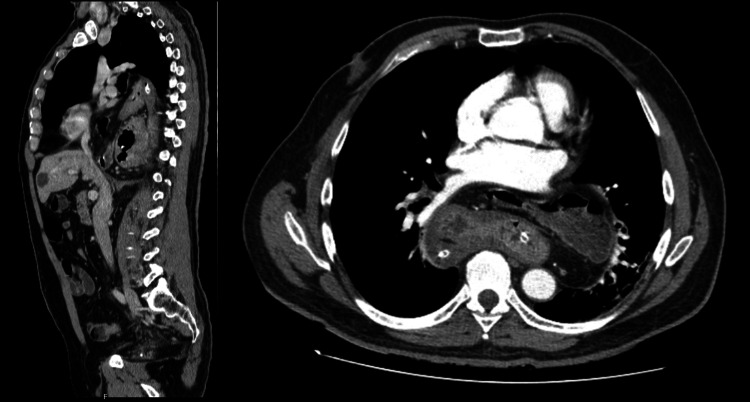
Computed tomography (sagittal and transverse section) performed to document a giant HH and to plan a treatment approach Giant HH with herniation of the fundus and gastric body (maximum intrathoracic transverse diameter of 165 mm), without evidence of volvulus, parietal thickening or signs of ischemia, and transverse colon; the neck of the hernia has a maximum transverse diameter of 43 mm.
